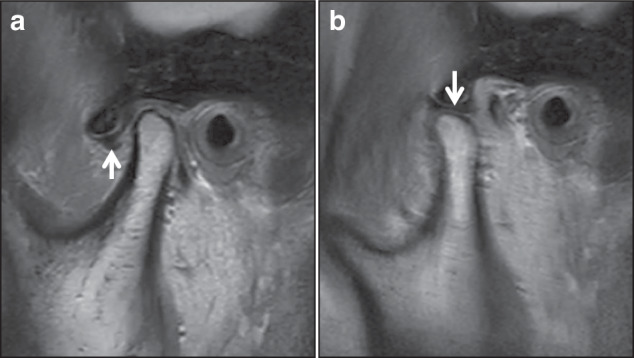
MRI PDWI imaging of anterior disc displacement with reduction, (a): in closed mouth position, (b): in open mouth position
